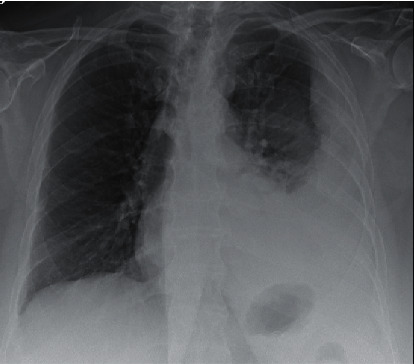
Portable upright chest radiograph, demonstrating loculated left-sided effusion with associated infiltrate and lobar pneumonia.
